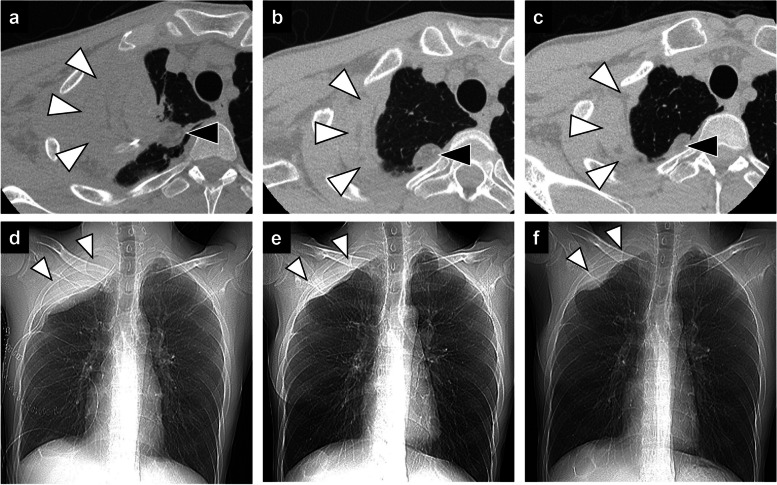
Chest CT follow-up of muscle flap and fat pad. Axial CT (A, B, C) and chest radiographs (D, E, F) at 7 days, 1 month, and 10 months postoperatively. The pedicled latissimus dorsi muscle flap (white arrowheads) and free fat pad (black arrowheads) gradually shrank with lung re-expansion but still filled the thoracic and pulmonary cavities, and the dead space did not recur postoperatively
